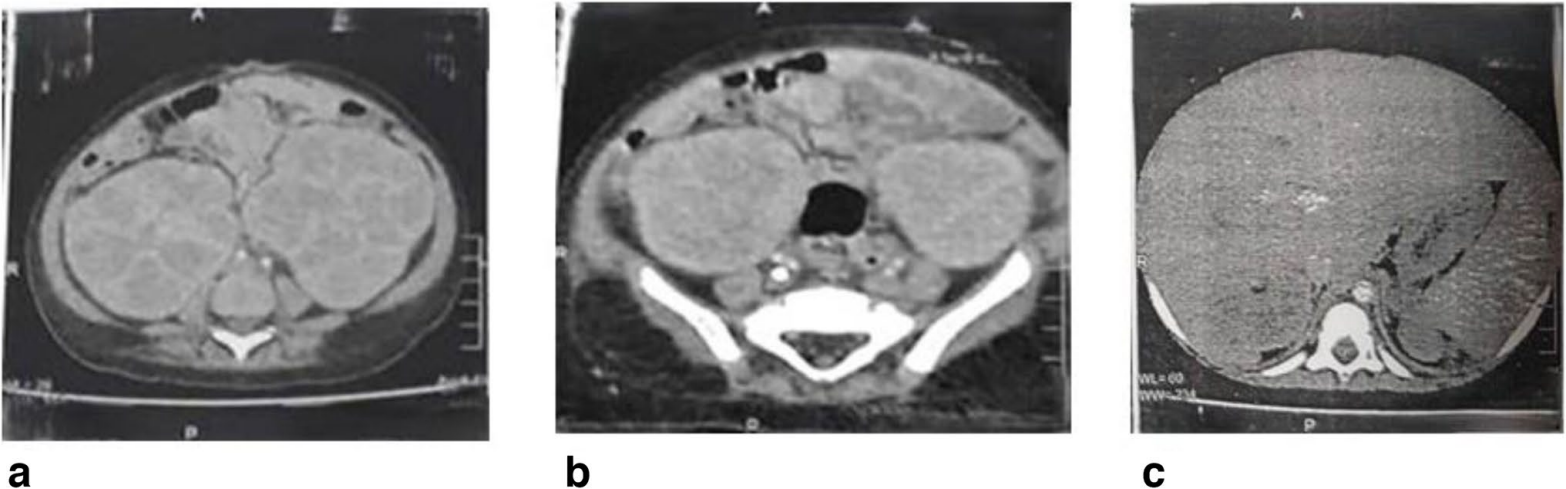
a CT images of abdomen and pelvis with IV contrast showing swollen renal parenchyma with cortical enhancement. b Bilateral kidney enlargement that extends down to sacroiliac joint with preserved kidney outlines. c CT scan showing diffusely enlarged liver and spleen. CT, computed tomography
